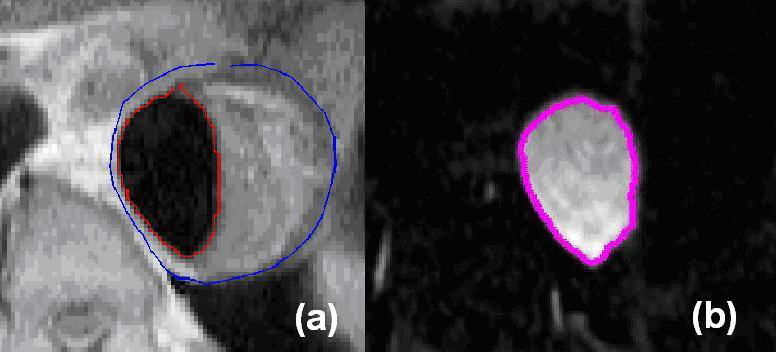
Image segmentation samples. Examples of the MR images and segmented contours of Set A1 (figure 1a) and Set A2 (figure 1b) for patient A.
